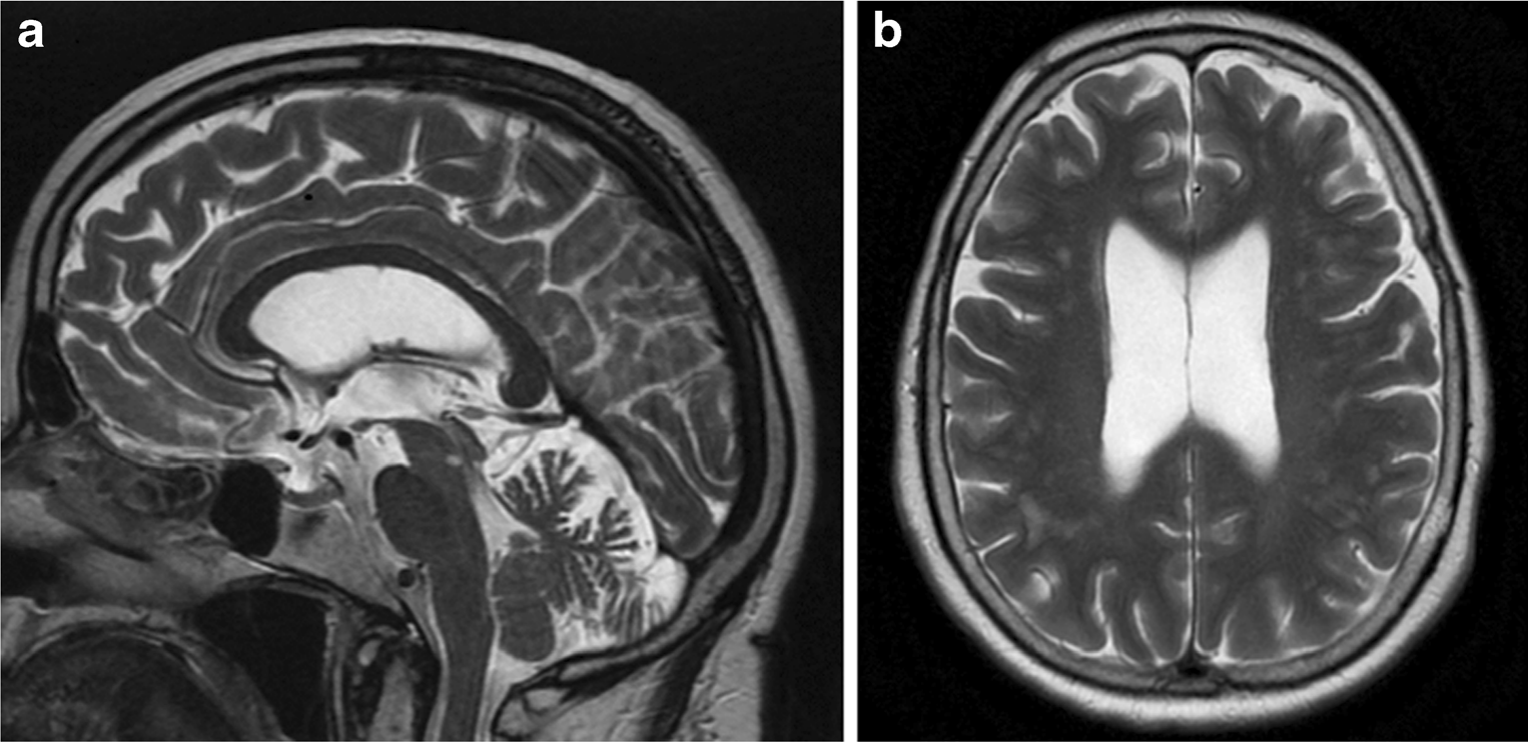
Brain MRI of patient III:2. a Mild vermis atrophy is evident on this midsagittal T2-weighted image. b Coronal T2-weighted image displays deep white matter hyperintensities
